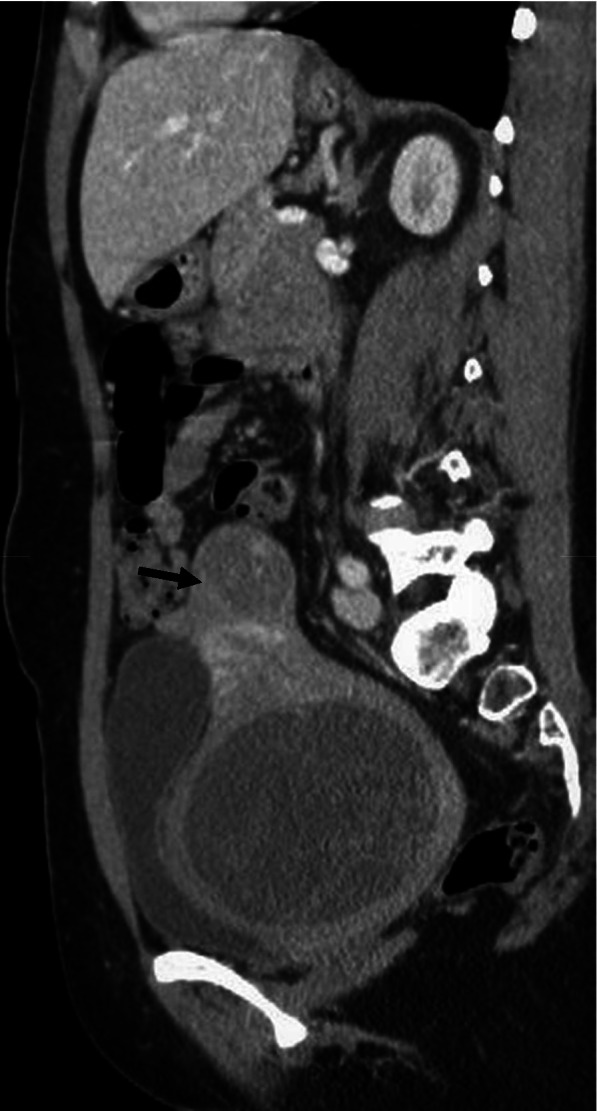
Sagittal reformatted CT image (iv contrast, portal phase). Large hypoattenuating well delineated mass located in the cervicovaginal area. There is a subserosal leiomyoma on the uterine fundus (arrowhead) and the endometrial cavity is not dilated. Bladder and rectal wall are not infiltrated by the mass
